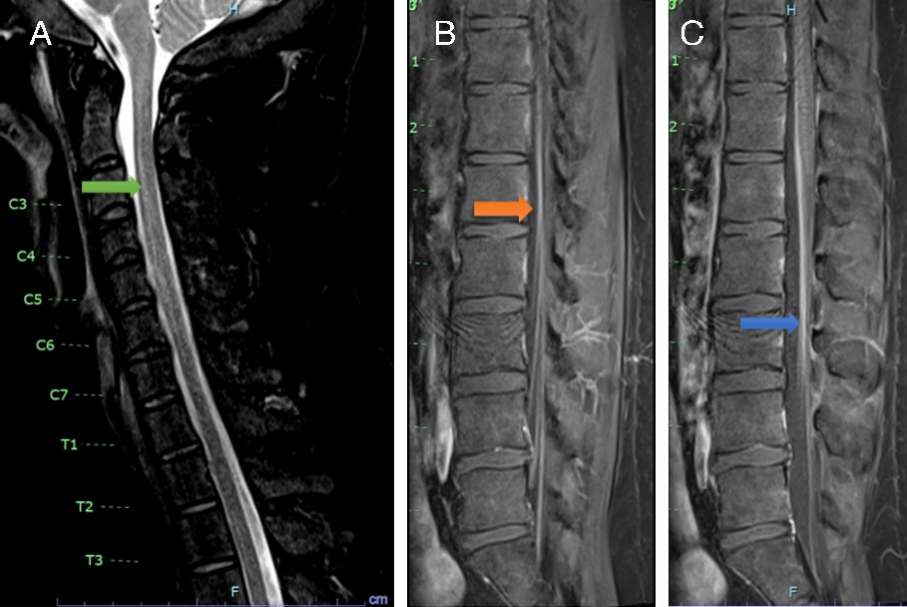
Spinal MRI. (A) MRI spine T2 sequences showing grey matter high T2 signal (green arrow). (B and C) Lumbosacral contrast-enhanced T1 MRI sequences showing smooth enhancement of the ventral surface of the cord (orange arrow) and of the cauda equina nerve roots (blue arrow).
